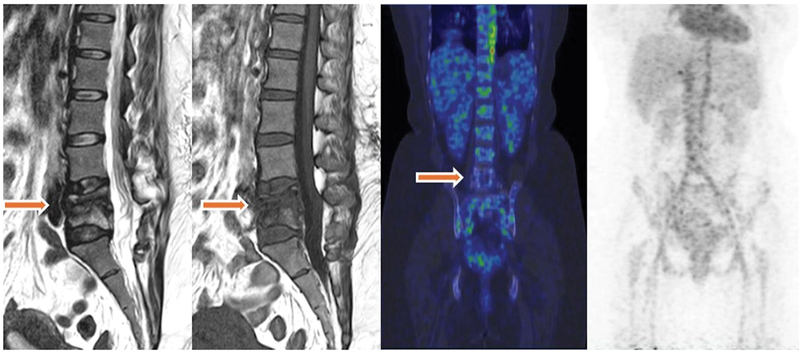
A 33-year-old lady with history of spinal tuberculosis involving L4-L5 vertebrae, came for end-of-treatment response after 2 years of antitubercular therapy (ATT) for spinal tuberculosis was symptom free. A Ga-68 positron emission tomography/computed tomography (PET/CT) citrate scan was performed to decide whether to stop ATT. The patient initially underwent a magnetic resonance imaging (MRI) scan for residual inflammatory changes and residual edema involving the L4-L5 vertebral bodies. A Ga-68 citrate PET/CT scan was performed 2 days later, which showed no tracer uptake in the previously involved region.
